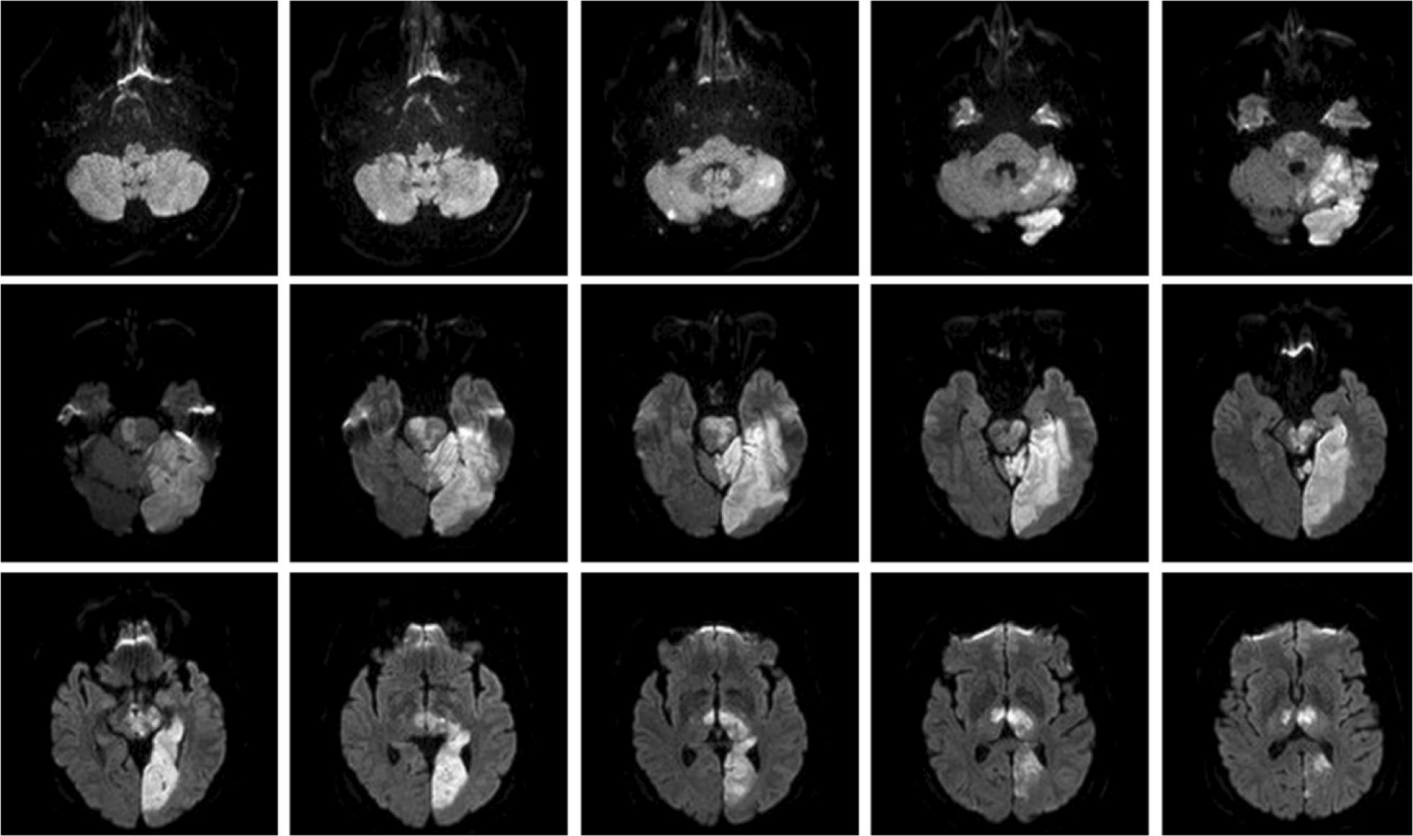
MRI scan of fictional patient Mr. M. presenting defects following a basilar artery embolism as part of a case vignette aimed at neurologists
